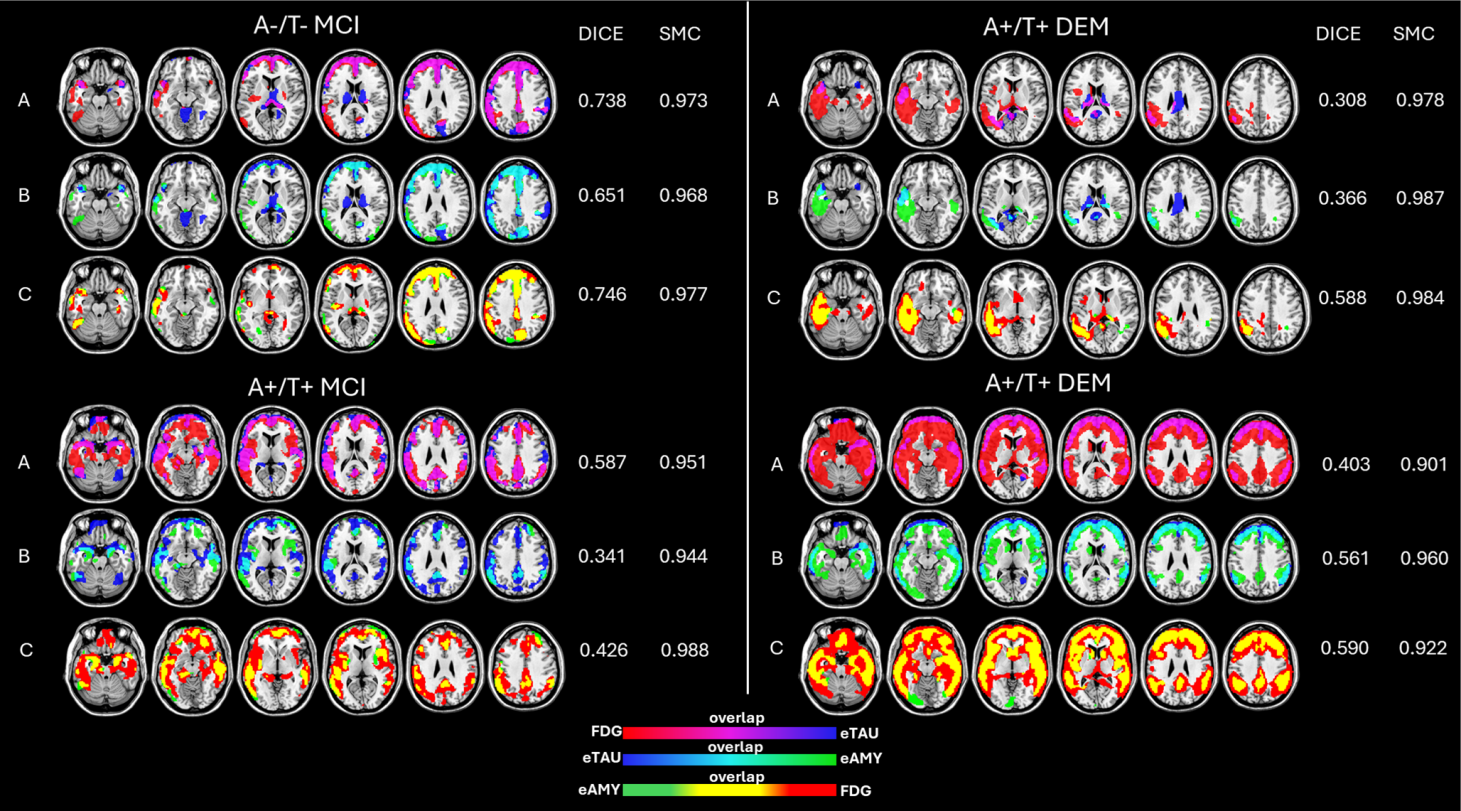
Individual examples of hypometabolic and hypoperfusion patterns at the single-subject level. Hypometabolism maps, two hypoperfusion maps (eTAU and eAMY), and their overlap were imposed on standard Montreal Neurological Institute template. These maps were obtained from the binarization of single-subject eTAU SPM T-maps, ^18^F-FDG PET SPM T-maps, and eAMY SPM T-maps (*p* < 0.05 uncorrected, k > 100). The Dice similarity index and the Simpe Matching coefficient (SMC) are reported to the right of the brain template of each subject for each modalities’ comparison. For each individual case, three rows (A, B, C) show the topographical comparison between eTAU and ^18^F-FDG PET (A), between eTAU and eAMY (B), and between ^18^F-FDG PET and eAMY (C). The reported individual cases are at different clinical disease stages and have different biomarker status. The first case (left) is A-/T- and shows FTD-like patterns. The other cases are all A+/T + with AD-like patterns. A = amyloid; AD = Alzheimer’s disease; DEM = dementia; eAMY = early phase amyloid-PET; eTAU = early phase tau-PET; FTD = frontotemporal dementia; MCI = mild cognitive impairment; SMC = simple matching coefficients; T = tau
